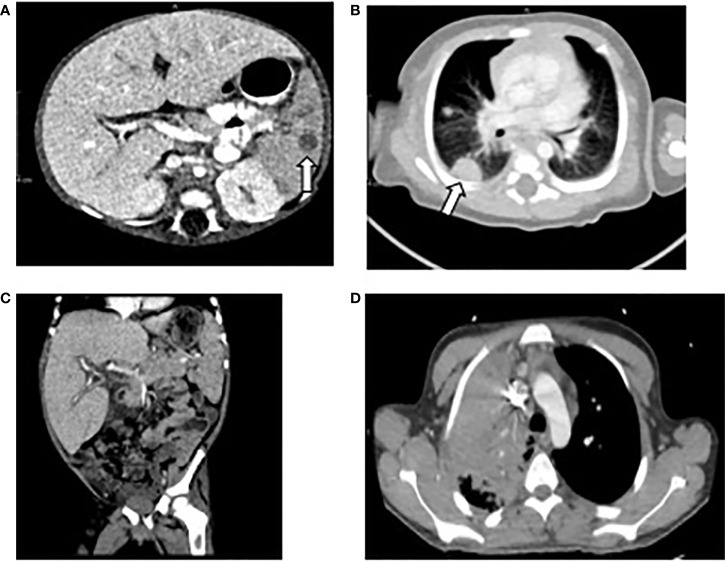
Computed Tomography of chest, abdomen, and pelvis of patients 1, 2, and 4. (A) Patient 1: splenic nodule indicated by arrow. (B) Patient 1: right sided pulmonary lesion indicated by arrow. (C) Patient 2: hepatosplenomegaly. (D) Patient 4: right upper lobe pulmonary lesion.
